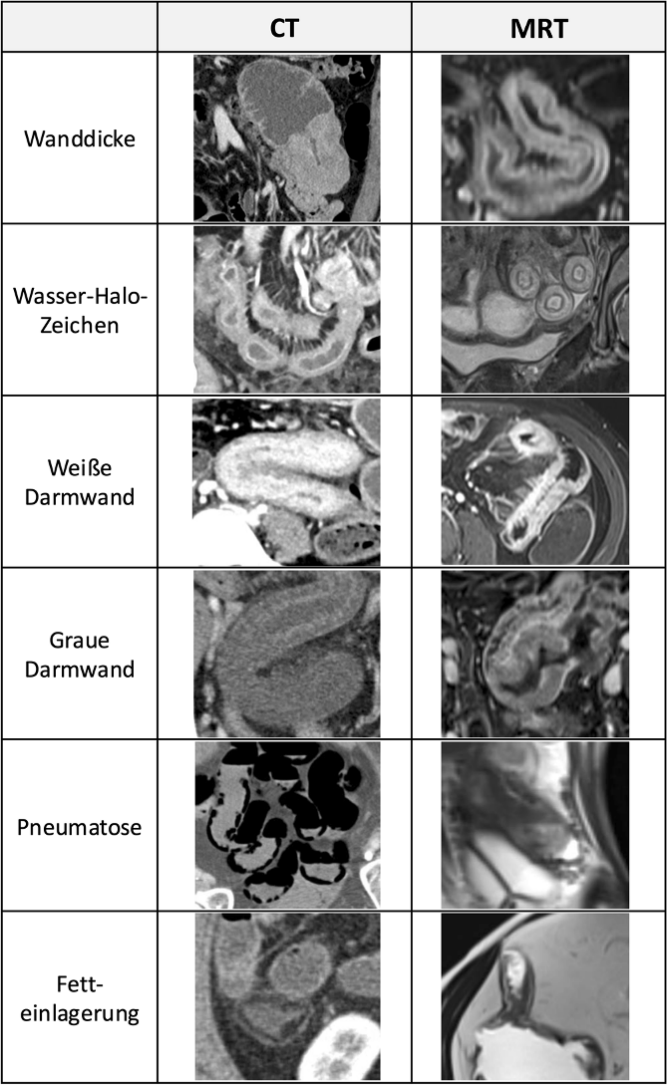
Darmwandmuster in der Computertomographie (*CT*) und in der Magnetresonanztomographie (*MRT*)
